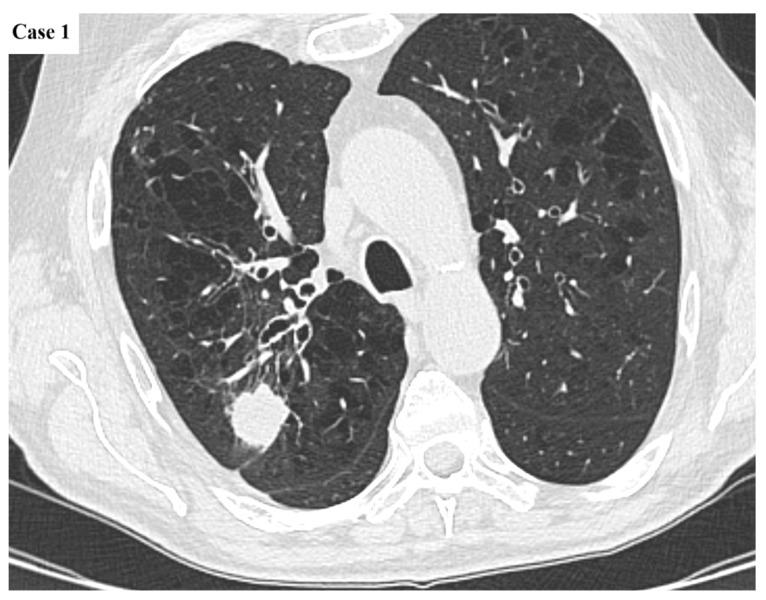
Axial CT image of a 26 mm lobulated mass located in the posterior segment of the right upper lobe. There is minimal tethering to the pleural surface. The large airways exhibit mild wall thickening and varicose bronchial dilatation, whereas the background lung parenchyma presents with diffuse centrilobular emphysematous changes.
